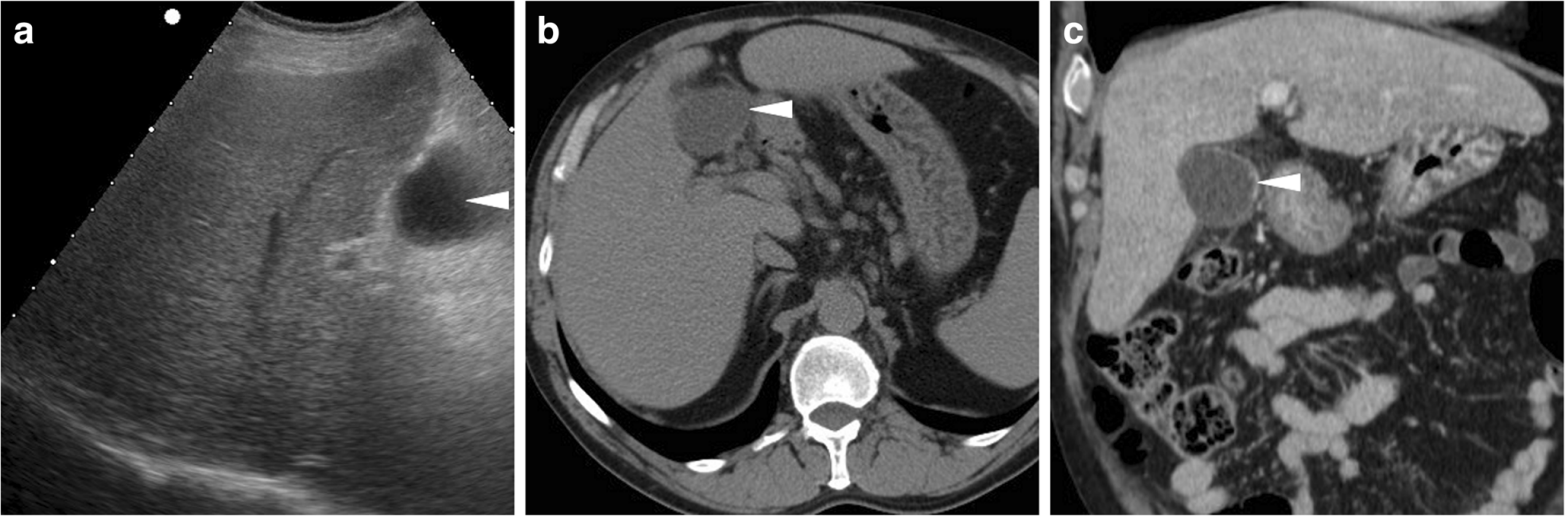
Mimicking a postoperative collection, a fluid-containing structure (*arrowheads*) with thin walls is seen 2 months after surgery in the operated gallbladder fossa at both ultrasound (a) as well as precontrast (b) and enhanced (c) CT, which corresponded to the gallbladder remnant after subtotal open cholecystectomy for acute cholecystitis and cholecysto-duodenal fistula
